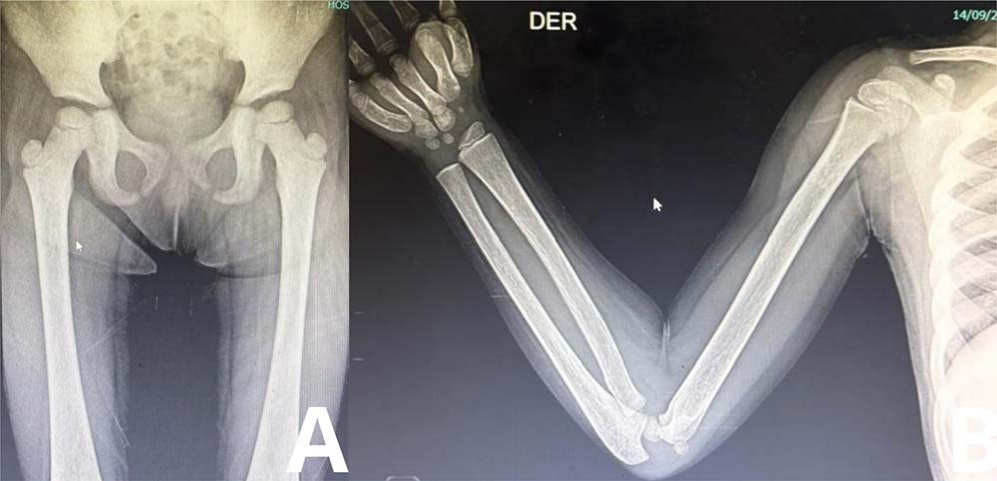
Radiographic evaluation of axial and appendicular skeleton (A-B). A. Anteroposterior radiograph of the lower abdomen and thighs showing multiple well-defined osteolytic lesions affecting the femurs bilaterally, with a geographic pattern and narrow transition zones. Mild cortical thinning is observed, particularly in the distal femoral metaphyses, suggestive of chronic LCH involvement. B. Anteroposterior radiograph of the right arm demonstrating a lytic lesion in the mid-diaphysis of the humerus with preserved cortical contour and no periosteal reaction, consistent with a non-aggressive process typical of LCH.
